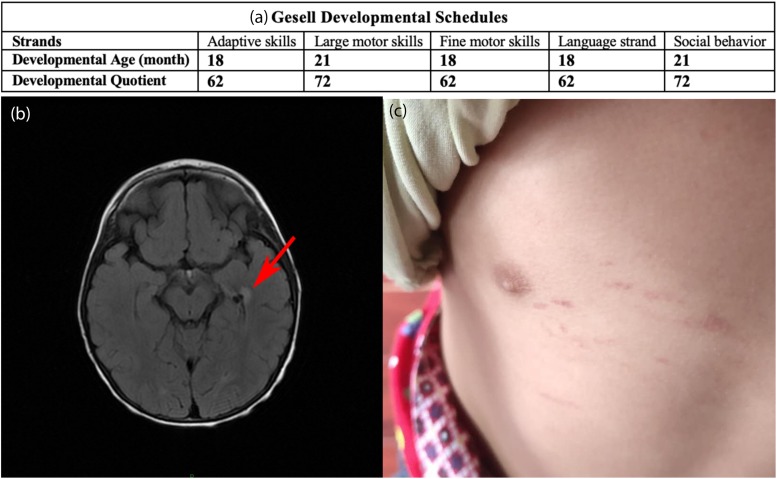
(a) Results based on the Gesell Developmental Schedules. Chronological age is 29 months. Developmental quotient<75 indicates developmental delay. (b) Brain MRI. The red arrow indicates shadows in the temporal horn of the left lateral ventricle. (c) Petechiae on the skin caused by falling appeared on the patient.
